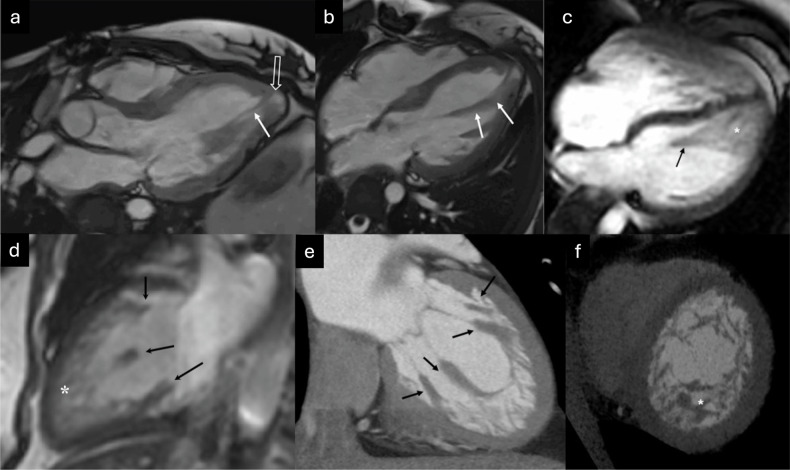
Papillary muscle anatomic variants. CMR (a SSFP three-chamber cine; b SSFP four-chamber cine; and c SSFP four-chamber cine) shows apical insertion of the posteromedial papillary muscle (solid arrows in a, b) in a patient with HCM. Note the small apical aneurysm of the LV (hollow arrow in a). c Apical insertion of the posteromedial papillary muscle in another patient (black arrow) generates apical ETs (asterisk). Accessory papillary muscle in a different patient is shown with CMR (d SSFP two-chamber view) and CT (e two-chamber view, f short-axis minimum-intensity projection) (arrows), associated with ET (asterisk)
